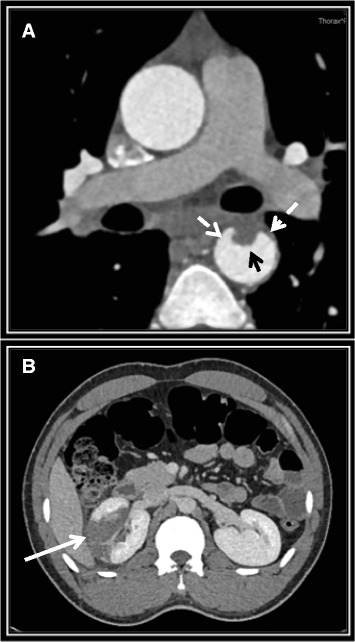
Computed tomography angiography of the chest and abdomen. It showed a non-circular thickening of the aortic wall of the descending thoracic aorta (dashed arrows) associated to an intra-luminal thrombus (*black solid arrow*) (a), and a right renal infarct (solid arrow) (b)
